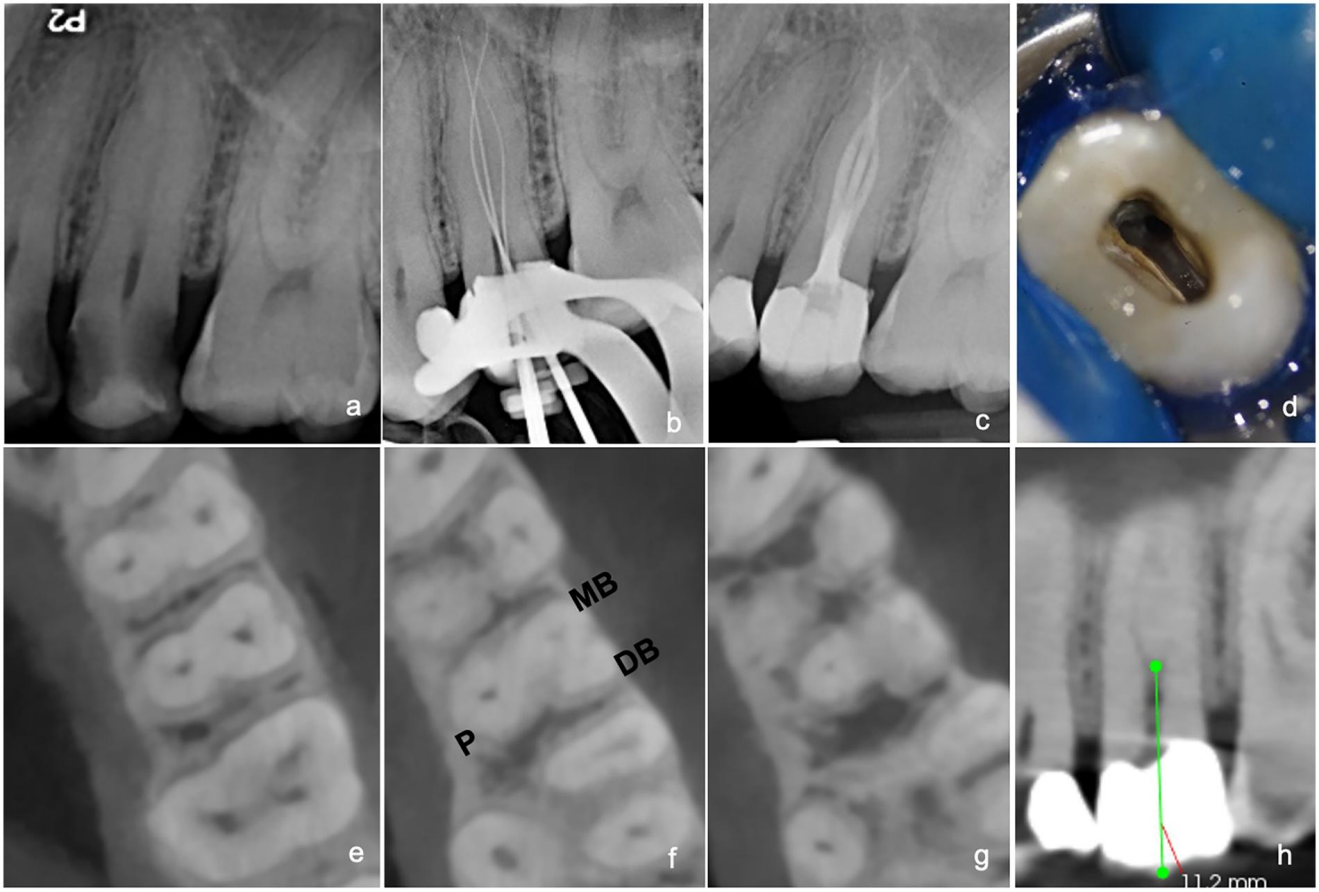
(a) Preoperative periapical radiograph of tooth #25. (b) Radiographic working length determination. (c) Postoperative radiograph. (d) Clinical image showing two buccal canal orifices. (e) Coronal CBCT view showing buccal and palatal canals. (f) Axial CBCT view at midroot level showing buccal canal bifurcation and three distinct roots. (g) Axial view showing apical confluence the confluence of buccal canals. (h) Sagittal CBCT view showing buccal canal bifurcation at midroot level.
